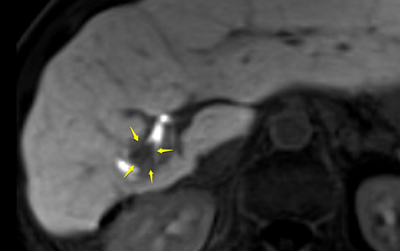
Hepatobiliary contrast (Gd-EOB-DTPA) MRI in the axial plane showed a nodular lesion in the right liver lobe, with low signal intensity compared with the remaining liver, associated with a filling defect in the adjacent bile duct (arrows).
